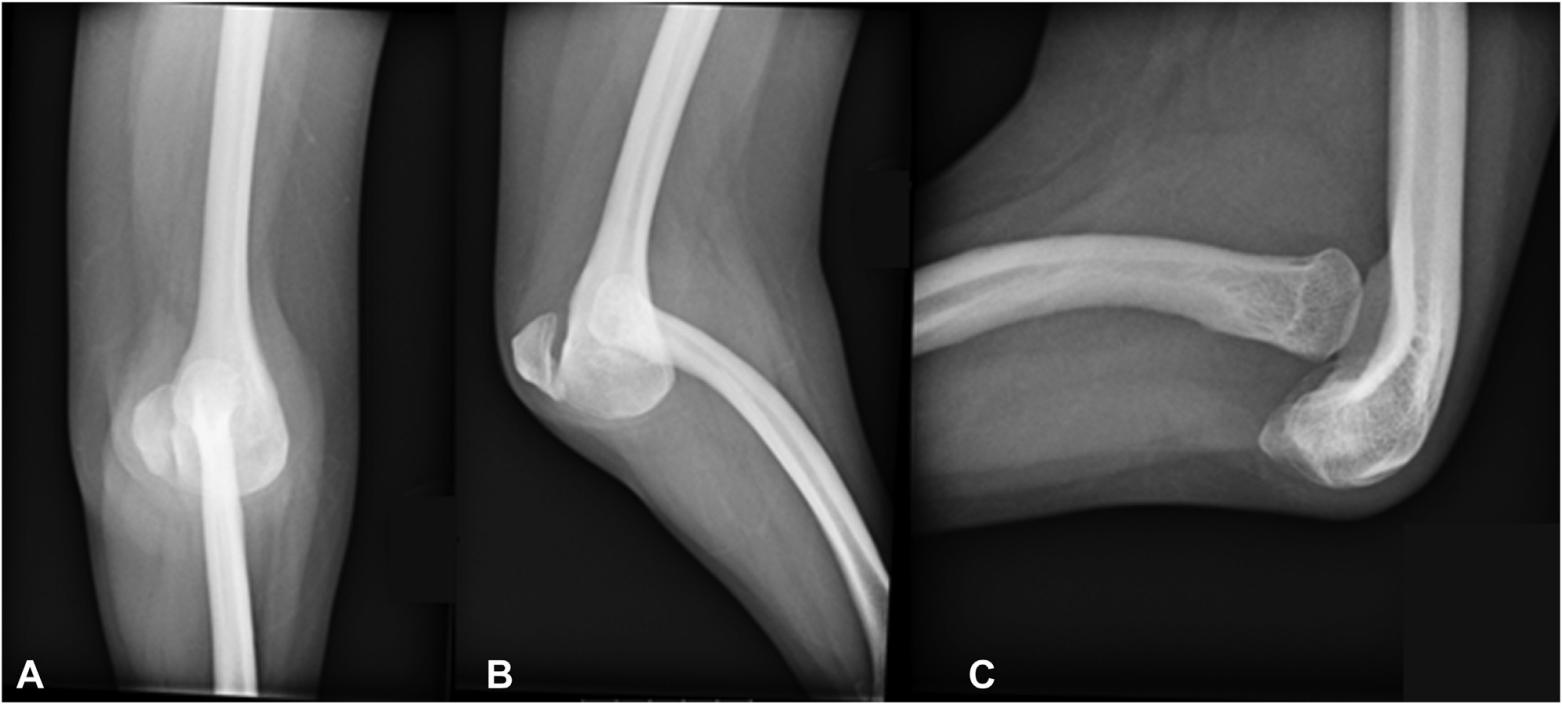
Left elbow radiographs. A Anteroposterior, B oblique, and C lateral radiographs of the left elbow demonstrate an abnormally developed elbow with an anteriorly dislocated, bowed radius forming a pseudoarticulation with the humerus and an abnormally rounded distal humerus. There is an ossific density near the distal humerus that is presumed to be the ulnar remnant.
